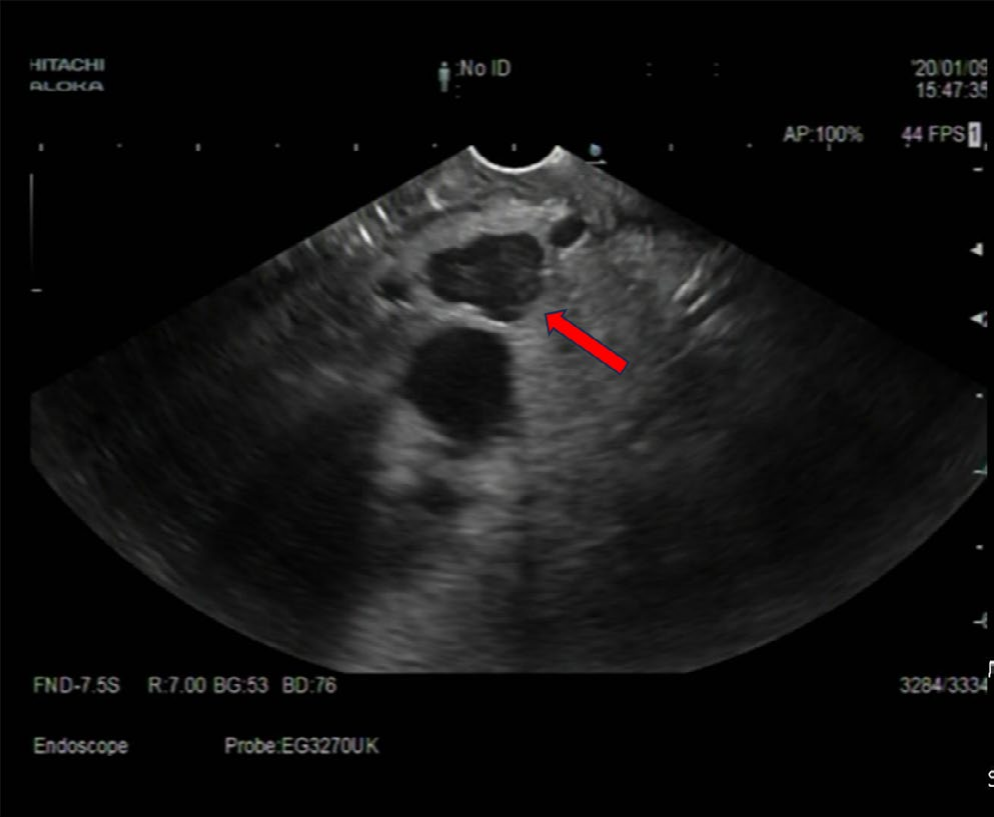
Tumor detected by endoscopic ultrasound.
